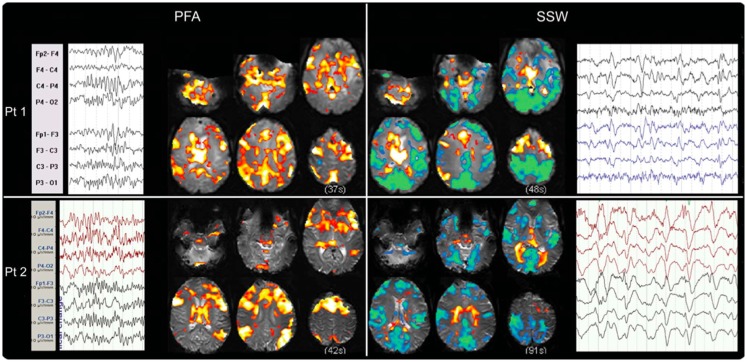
Electroencephalography–functional magnetic resonance imaging of generalized paroxysmal fast activity (GPFA) and slow spike-and-wave (SSW) in individual LGS patients is shown. In individual patients, GPFA and SSW produce different blood–oxygen-level-dependent (BOLD) response patterns. GPFA shows increased BOLD in diffuse association network regions, as well as brainstem, basal ganglia, and thalamus. SSW shows a different pattern, with decreased BOLD signal in primary cortical areas. The number of events in seconds, at the bottom of each panel, is the sum of the length of all individual epileptiform events recorded during the EEG for each patient. Pt, patient. Re-printed with permission from Pillay and colleagues (72).
